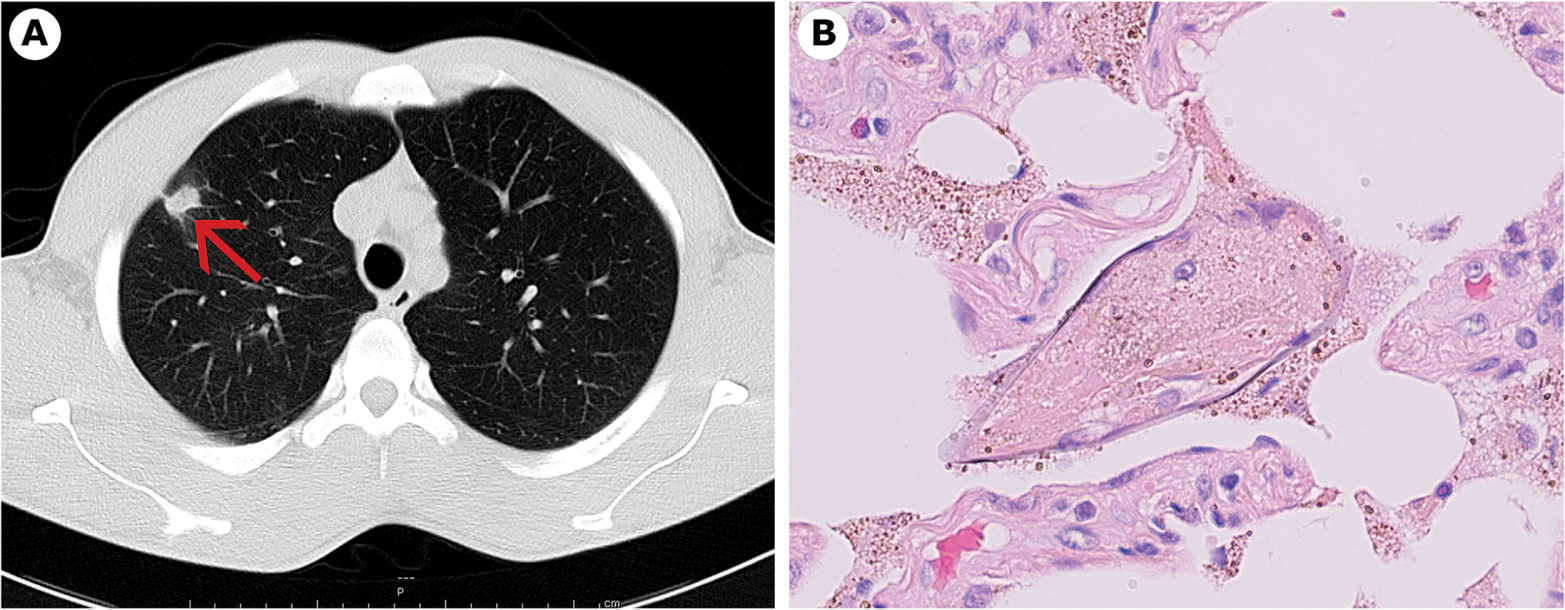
Case 2. a) Pulmonary nodule at CT scan. b) *S. mansoni* egg in lung tissue.
